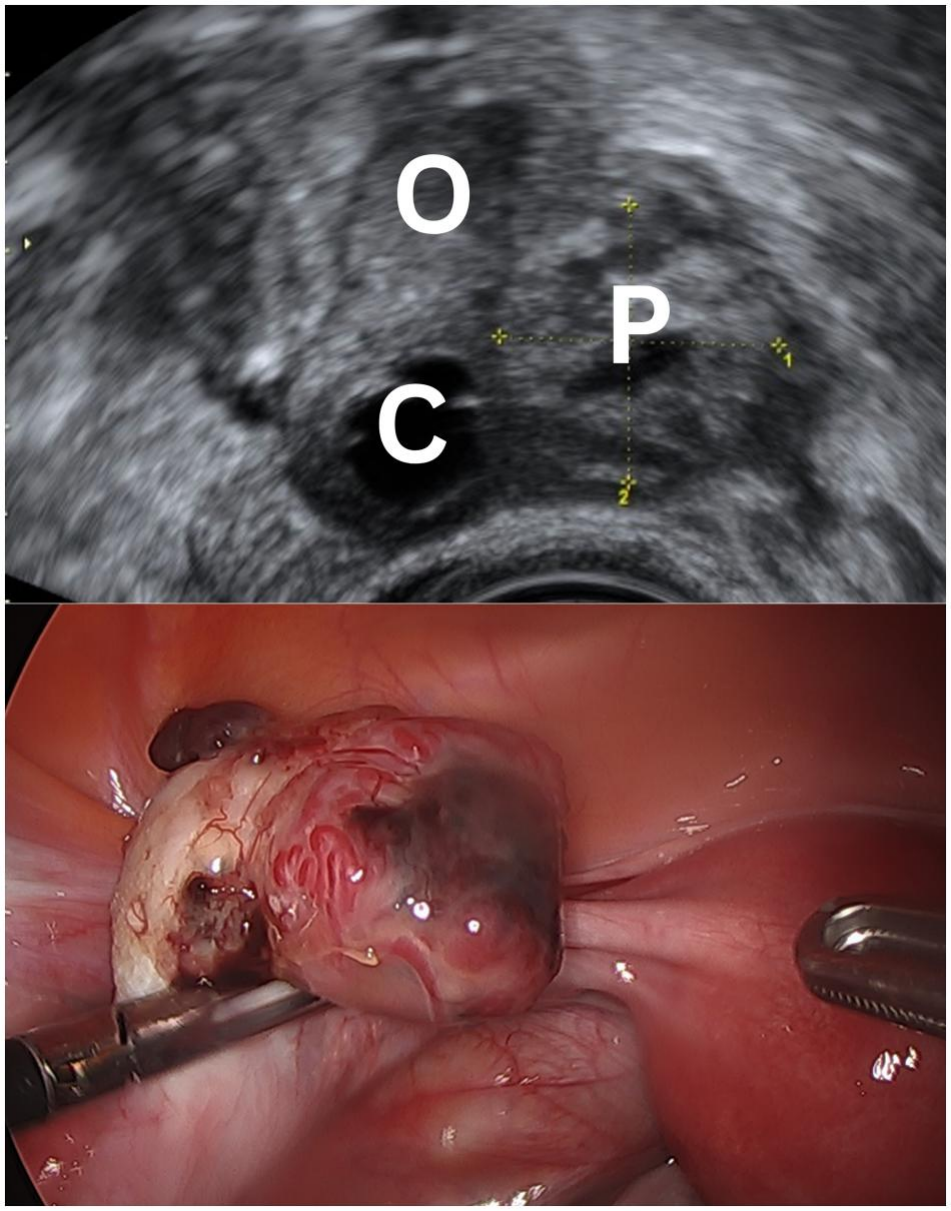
Ultrasound and laparoscopic images of an ovarian ectopic pregnancy. The ultrasound image demonstrates the ovary (O) containing a corpus luteum (C) and an ovarian ectopic pregnancy (P) with a defined hyperechoic trophoblast ring. At laparoscopy, the pregnancy can be seen protruding from the surface of the ovary. Resection of the ovarian ectopic was performed and the ovary was conserved.
